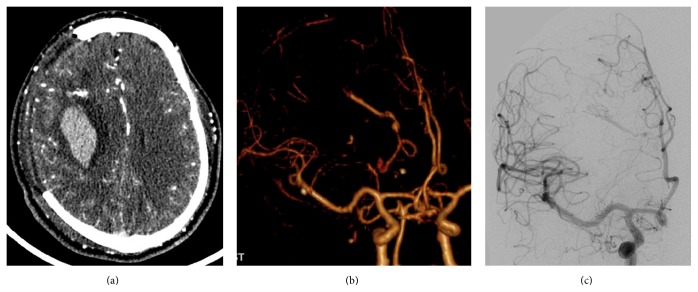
One case showed abnormal venous drainage in CTA. (a) Postoperative day 1 of decompressive craniectomy, enhanced thin layer CT scanning showing a tiny nidus of dense vessels in the front edge of hematoma. (b) VR reconstructed CTA images showing only abnormal venous drainage, no obvious nidus and feeding artery. (c) DSA confirmed micro-AVMs and feeding artery is a tiny branch of anterior cerebral arteries.
